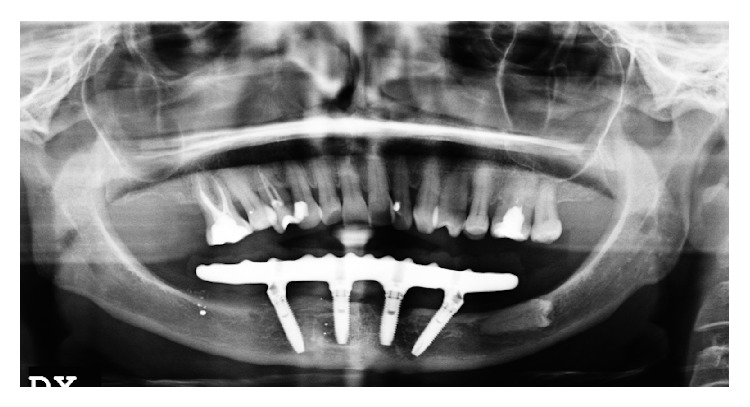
Orthopantomography of the patient after 36 months from surgery.
